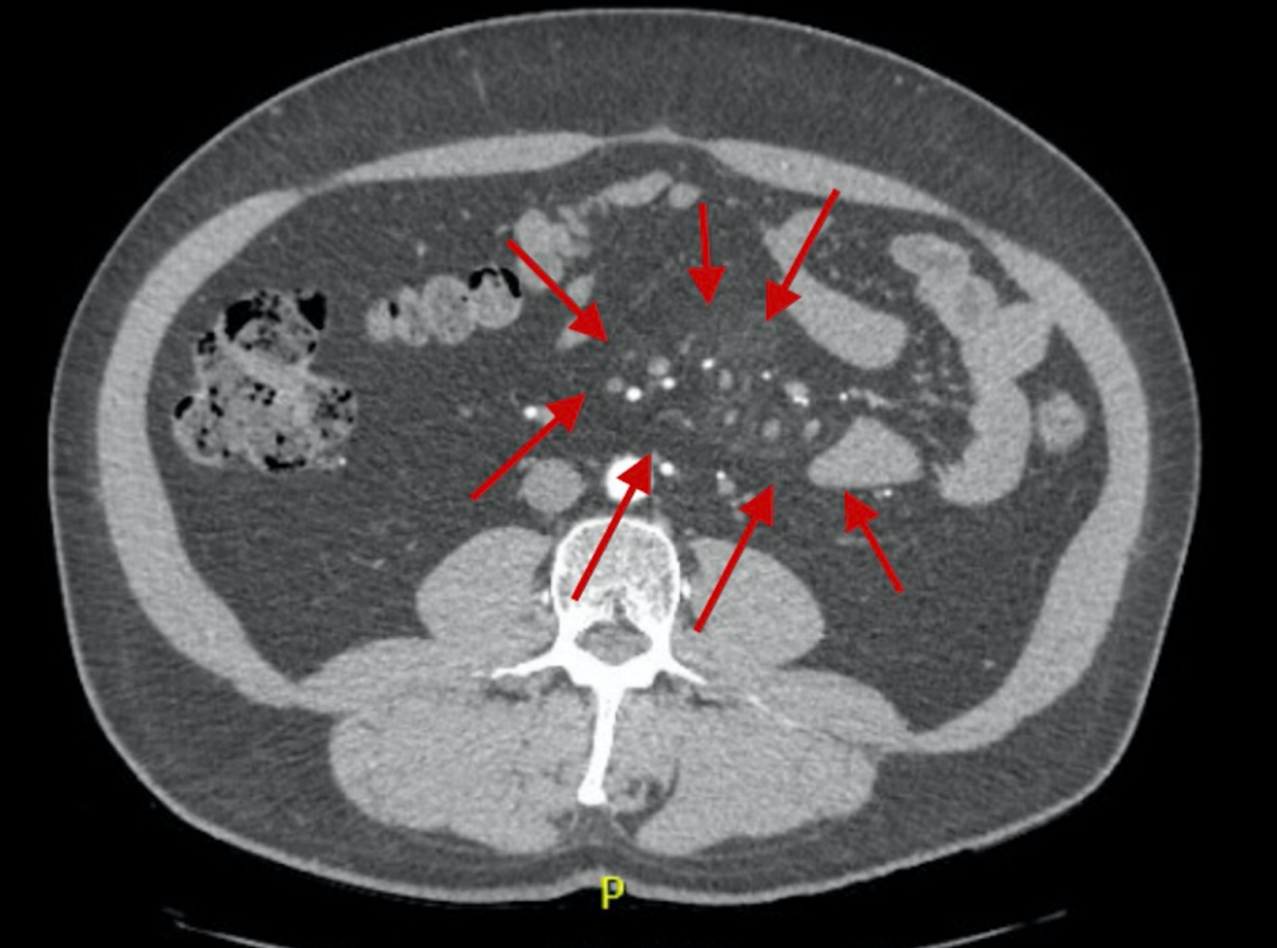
Computed Tomography of the Abdomen The red arrows denote the mesenteric fat stranding.
